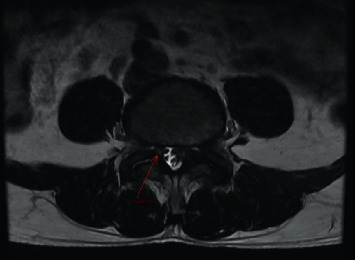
Preoperative magnetic resonance imaging (MRI) scan. This axial T2-weighted MRI image shows a cystic lesion (red arrow) arising from the right L4–5 facet joint, causing the narrowing of the right neural foramina. Bilateral facet synovial cysts are also evident.
